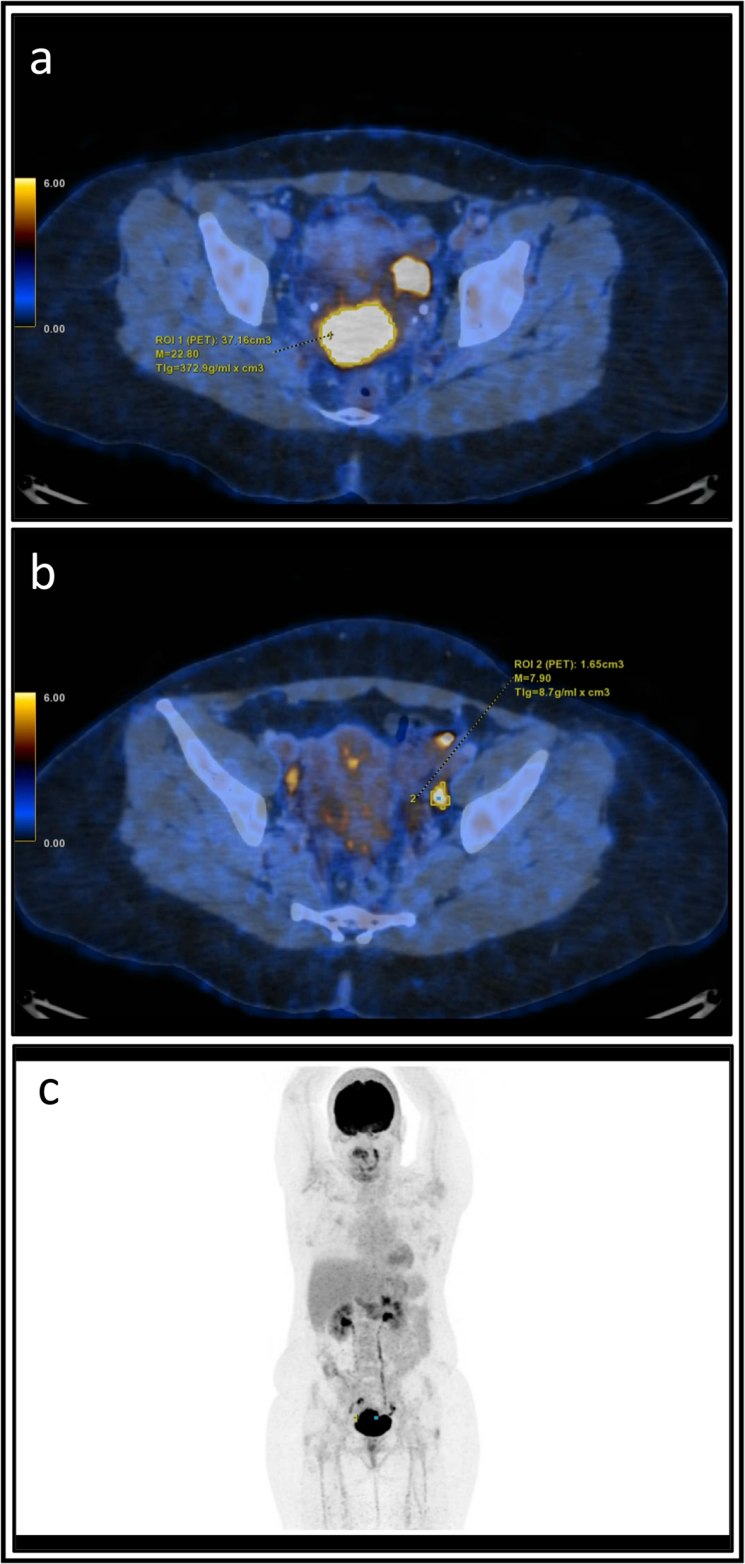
PET images of a patient presenting with a cervical tumor with left pelvic lymph node involvement. a) cervical tumor contouring with semi-automatic tumor thresholding method at 40% of the SUVmax (SUVmax 22.8; TLG 372.9 g/ml*cm^3^; MTV 37.16 cm^3^); b) the same procedure with a left pelvic involved lymph node with SUVmax 7.9; TLG 8.7 g/ml*cm^3^; MTV 1.65 cm^3^; c) Maximum intensity projection showing the absence of distant metastasis
